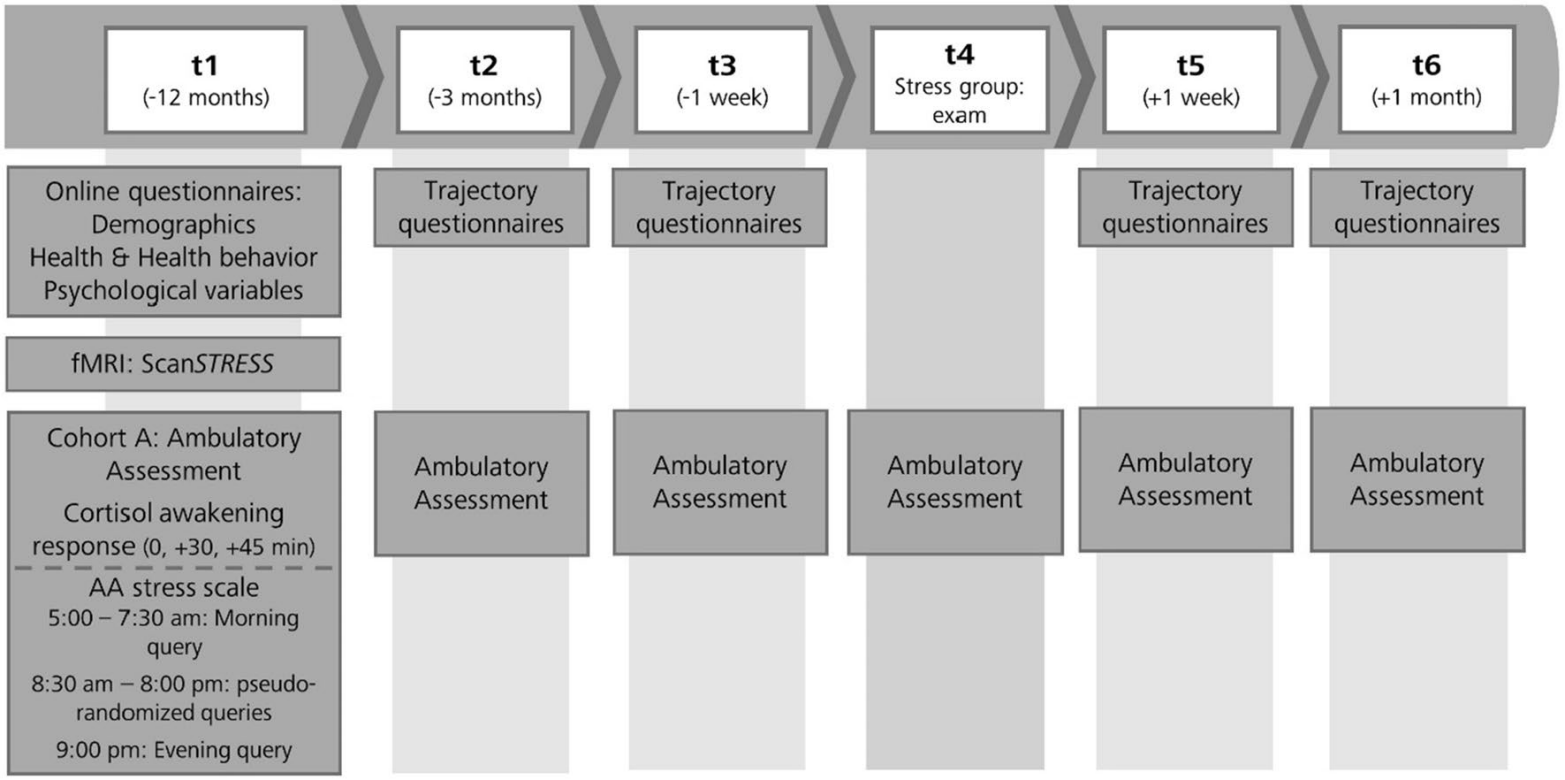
Timing of the data collection for the ambulatory assessment and the MRI measurement. For an overview of the entire study procedure of the LawSTRESS project see https://epub.uni-regensburg.de/51920/.
